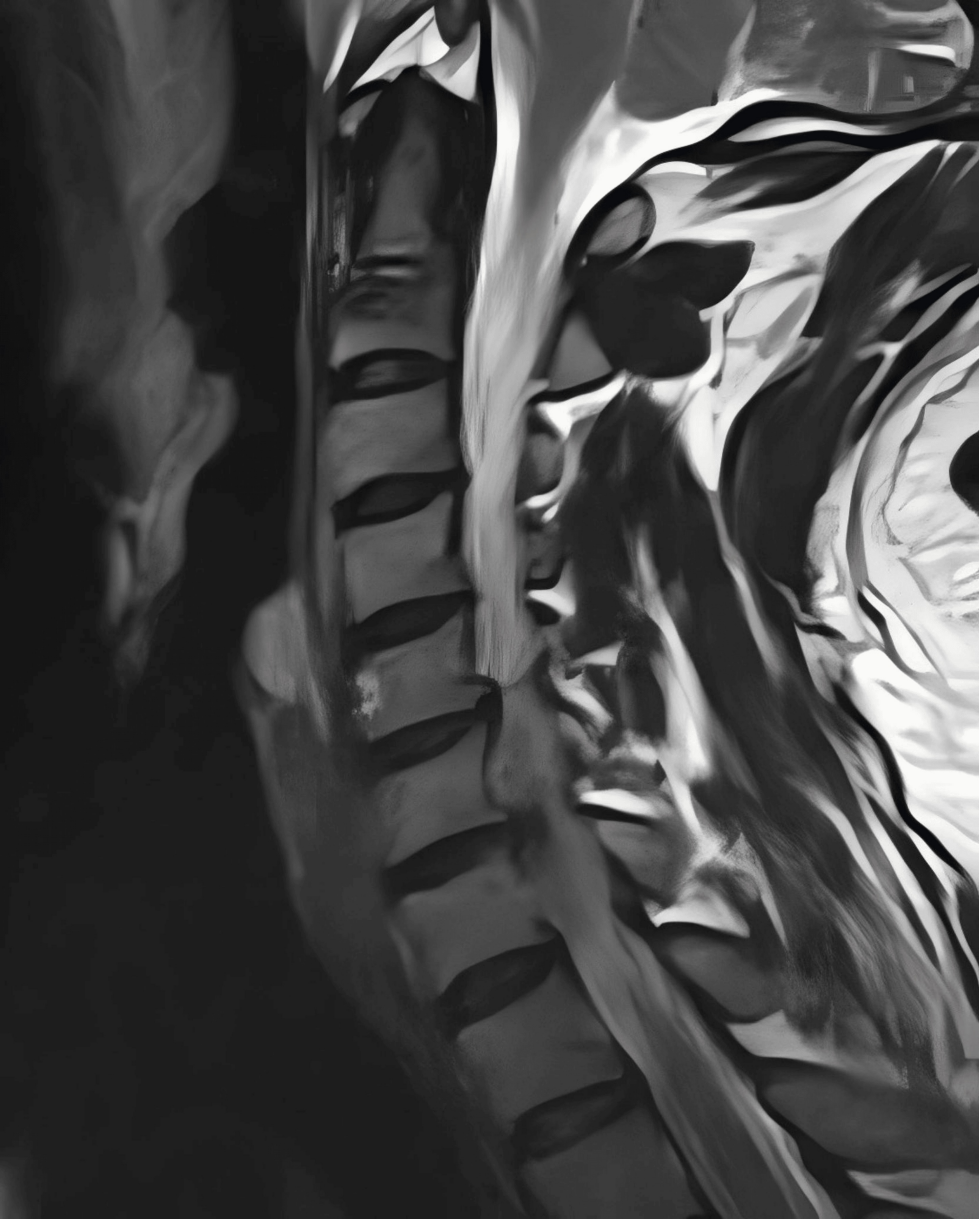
Patient's pre-operative magnetic resonance imaging (MRI) The patient's sagittal MRI shows a herniated disc at C6/7, compressing the nerves at the back
